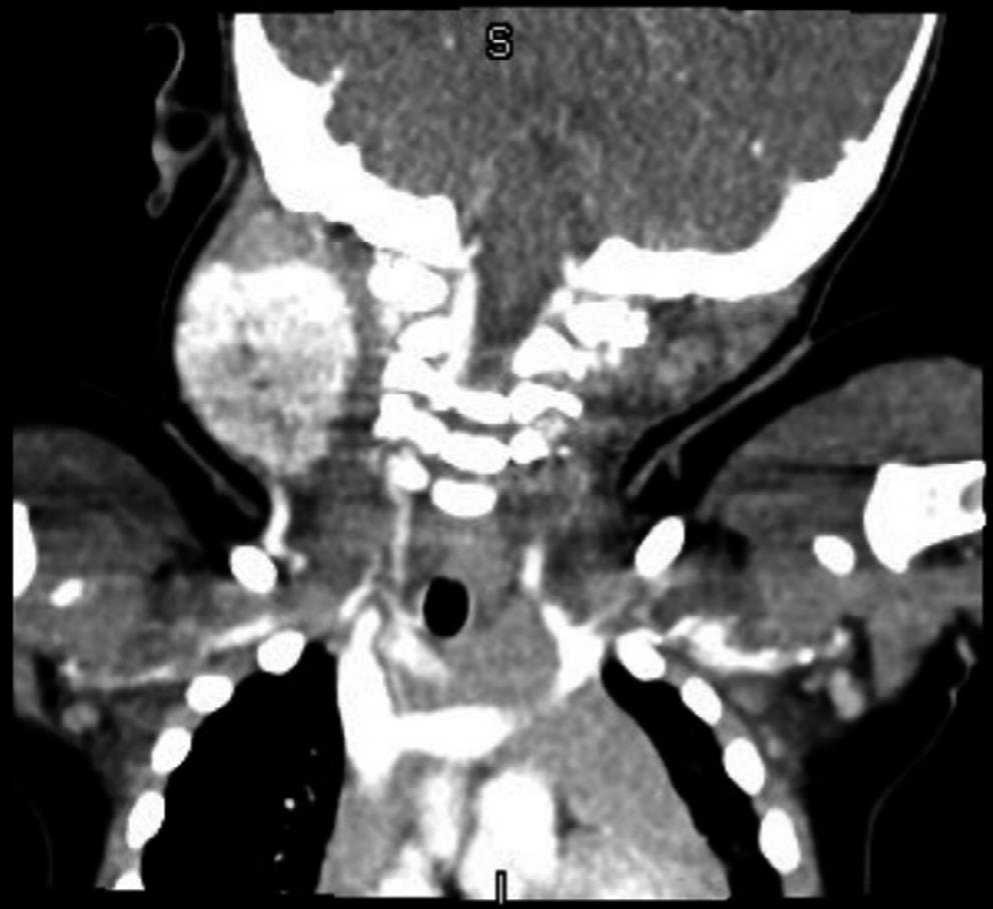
Prior CT demonstrating the size of the IH approximately 1 y prior
